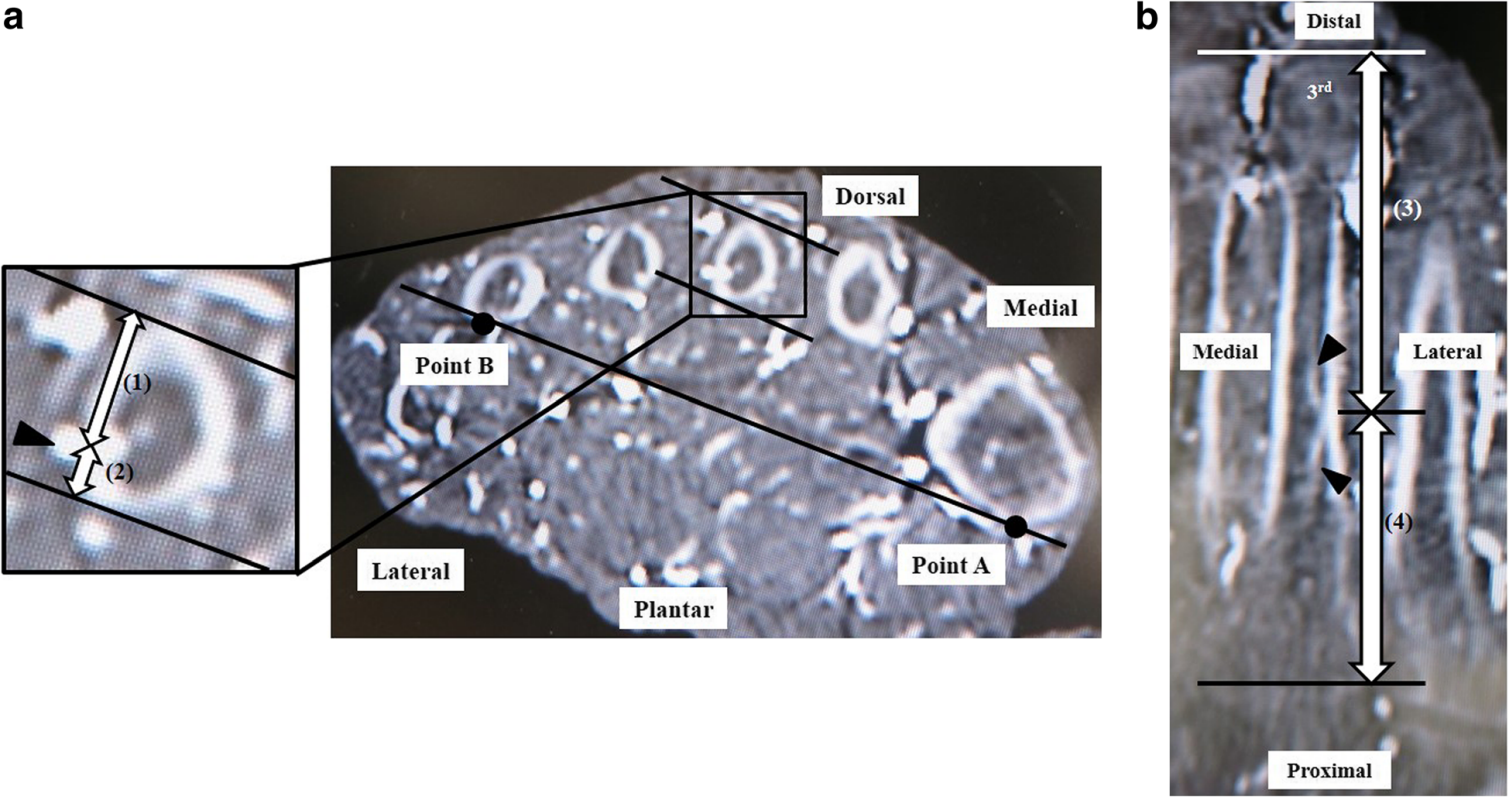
Enhanced CT images showing nutrient artery parameters in relation to the lesser metatarsals. a Distance from the dorsal plane parallel to a line between the most plantar point of the first metatarsal (point A) and fifth metatarsal (point B) to the point of entry of the nutrient artery into the lesser metatarsal (1) and the distance from a line between the most plantar point of the first metatarsal (point A) and fifth metatarsal (point B) to the point of entry of the nutrient artery into the lesser metatarsal (2) in the axial plane. b Distance from the distal epiphysis to the point of entry of the nutrient artery into the lesser metatarsal (3), the distance from the proximal epiphysis to the point of entry of the nutrient artery into the lesser metatarsal (4) in the coronal plane. The arrow head indicates the nutrient artery
